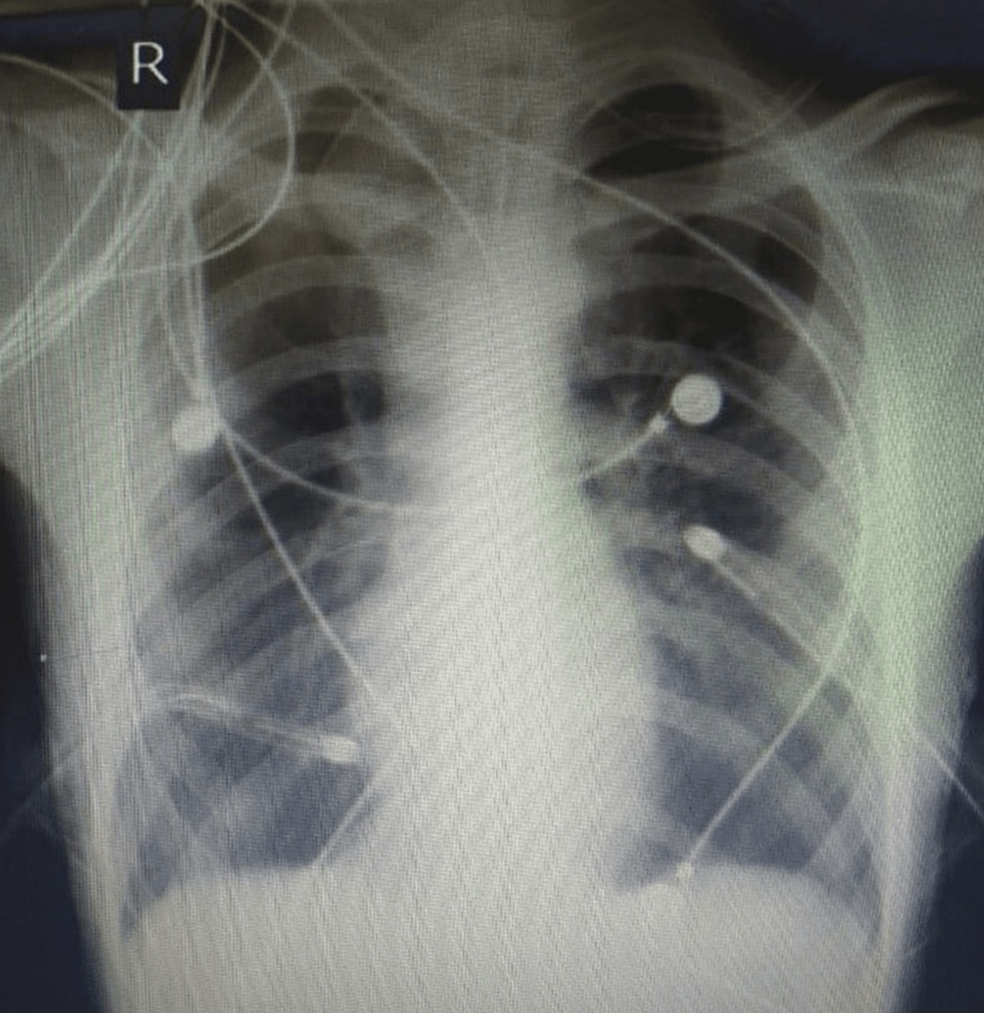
Post ICD insertion, the X-ray showed full expansion on the right side and to some extent, on the left side
